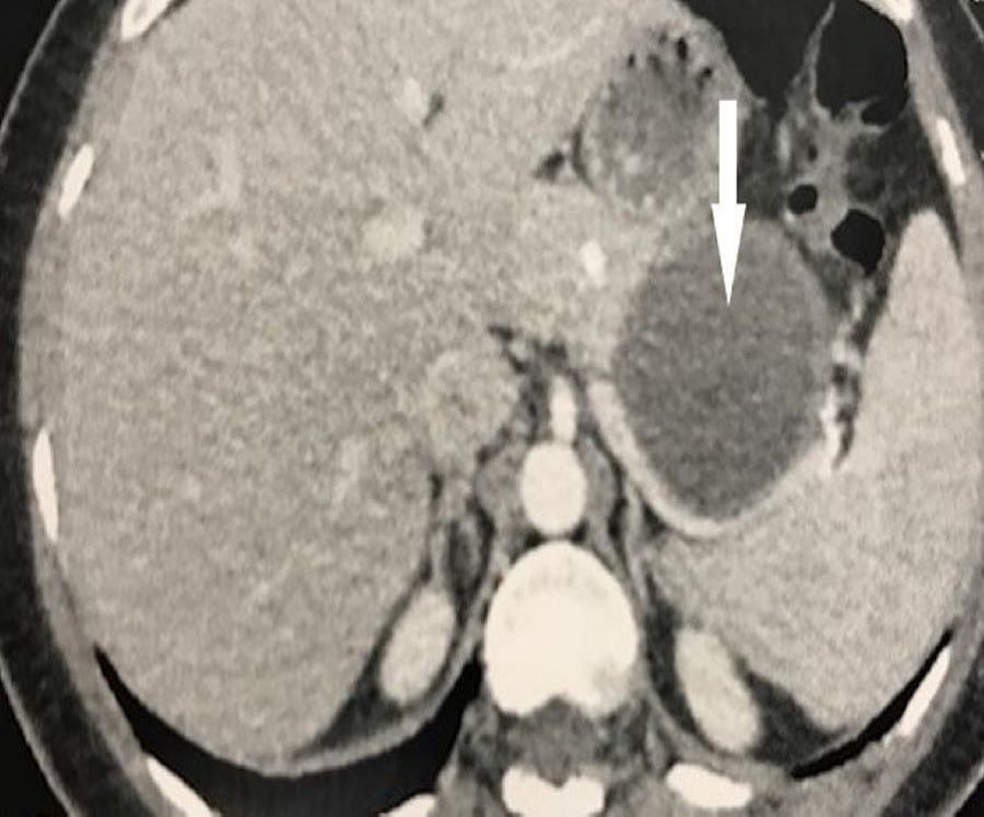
A uro-CT suggesting a cystadenoma of the tail of the pancreas. CT: computed tomography
